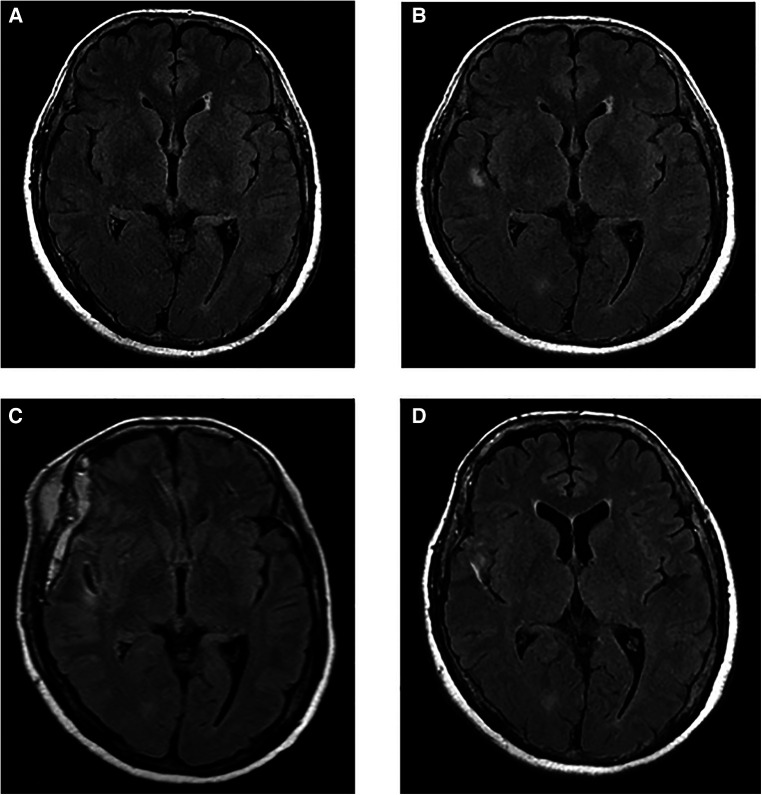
(A) MRI-FLAIR image taken six months before this admission. (B) MRI-FLAIR image taken at this admission. A FLAIR hyperintense lesion was detected in the inner temporal lobe. (C) MRI-FLAIR image taken at post-operative day 7. The FLAIR hyperintense lesion persisted. (D) MRI-FLAIR image taken eight months after discharge. The FLAIR hyperintense lesion disappeared.
